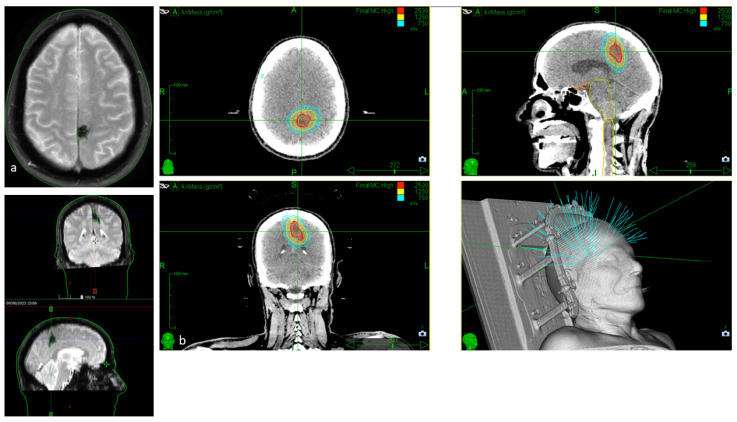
(a). Axial, coronal, and sagittal GRE T2*-weighted MRI of a 79-year-old patient with severe cardiologic comorbidities and a progressive motor and sensory deficit of the right leg and foot, showing a left parasagittal CM with typical blooming artifact. (b). SRS treatment planning of the same patient for whom surgery was considered too risky.
